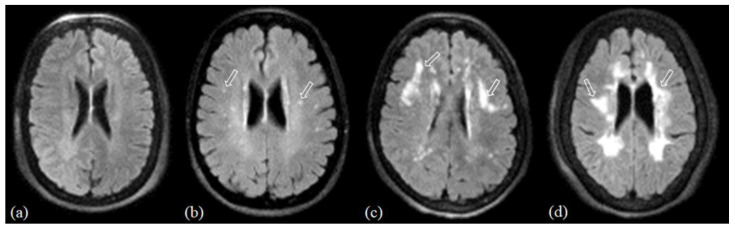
Representative axial fluid-attenuated inversion recovery (FLAIR) MR images showing increasing severity of white matter hyperintensities (WMHs; arrowed) with the corresponding assigned Fazekas grades. (a) No WMH, grade 0; (b) multiple small WMH, grade 1; (c) WMH with beginning confluence, grade 2; (d) diffuse confluent WMH, grade 3.
